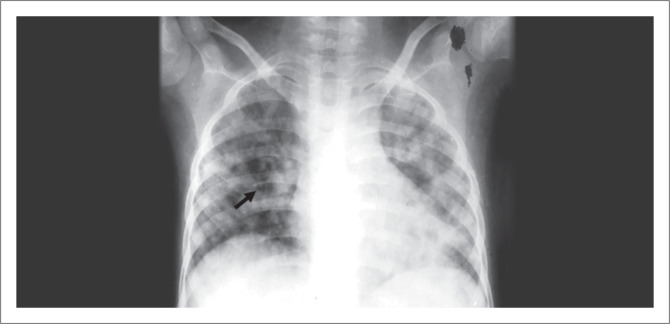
Chest radiograph of a 12-year-old female, shows bilateral air space opacities with few cystic lucencies in the right perihilar region (black arrow).
